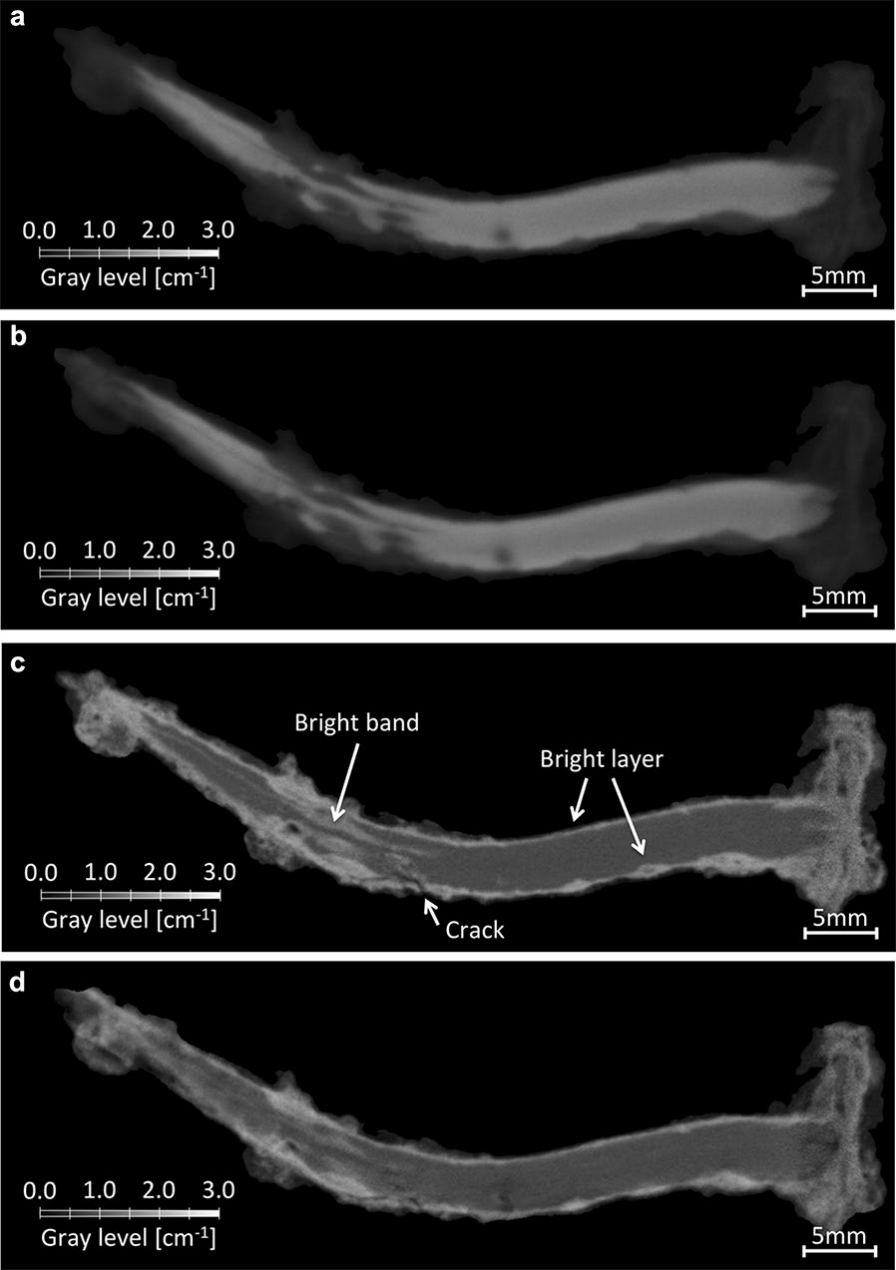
Tomography images obtained before and after treatment of nail B. There is no significant difference in the X-ray images (a and b), but some $$\approx 100$$
